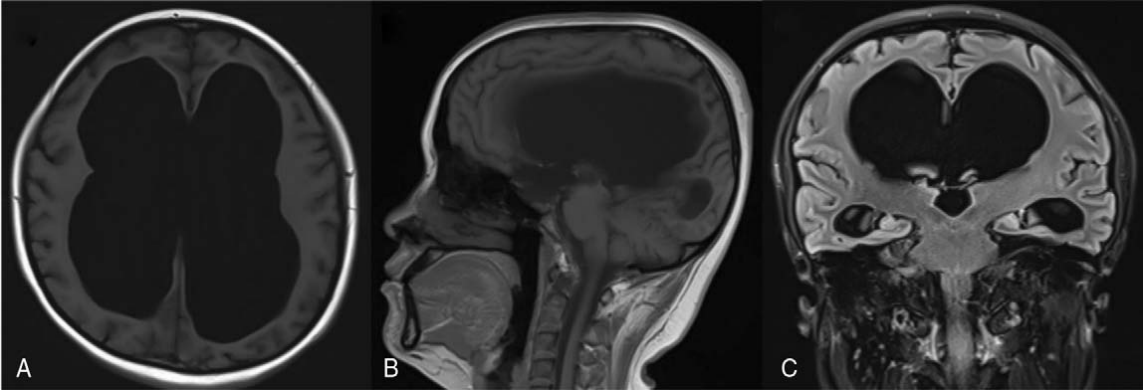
From left to right: T1-weighted magnetic resonance imaging in the transverse, coronal, and sagittal planes of the patient (A–C). Overt triventriculomegaly, cerebral aqueduct stenosis, and a concomitant severe ventriculomegaly are reported. Evans index: 0.66. Third ventricle width: 30 mm.
